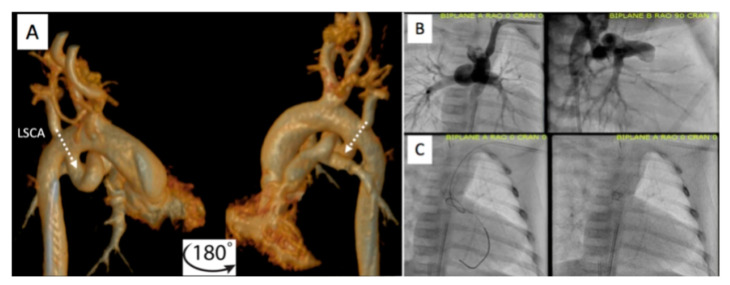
Additional example of pre-procedural CT imaging (A) aiding with access site selection for successful PDA stenting (B,C) via the left axillary artery (LAA) to the left subclavian artery (LSCA). Revised from ref [9].
